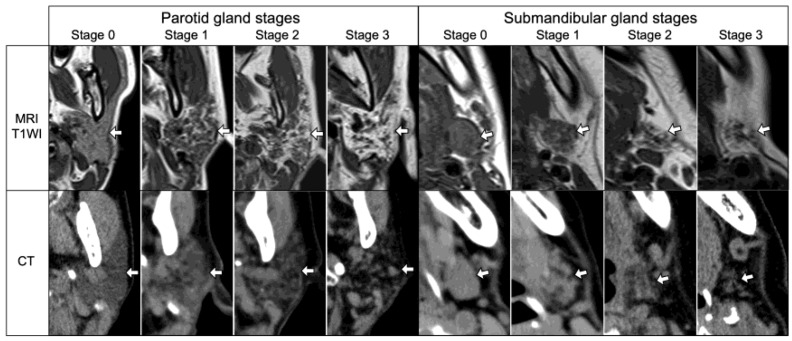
SS disease stage of parotid glands (white arrows) and submandibular glands (white arrows) on MRI and CT. Stage 0, no obvious fatty degeneration; Stage 1, weak fatty degeneration (up to 1/3 of the entire gland area); Stage 2, moderate fatty degeneration (more than 1/3 and up to 2/3 of the entire gland area); and Stage 3, severe fatty degeneration (over 2/3 of the entire gland area). T1WI, T1-weighted image.
